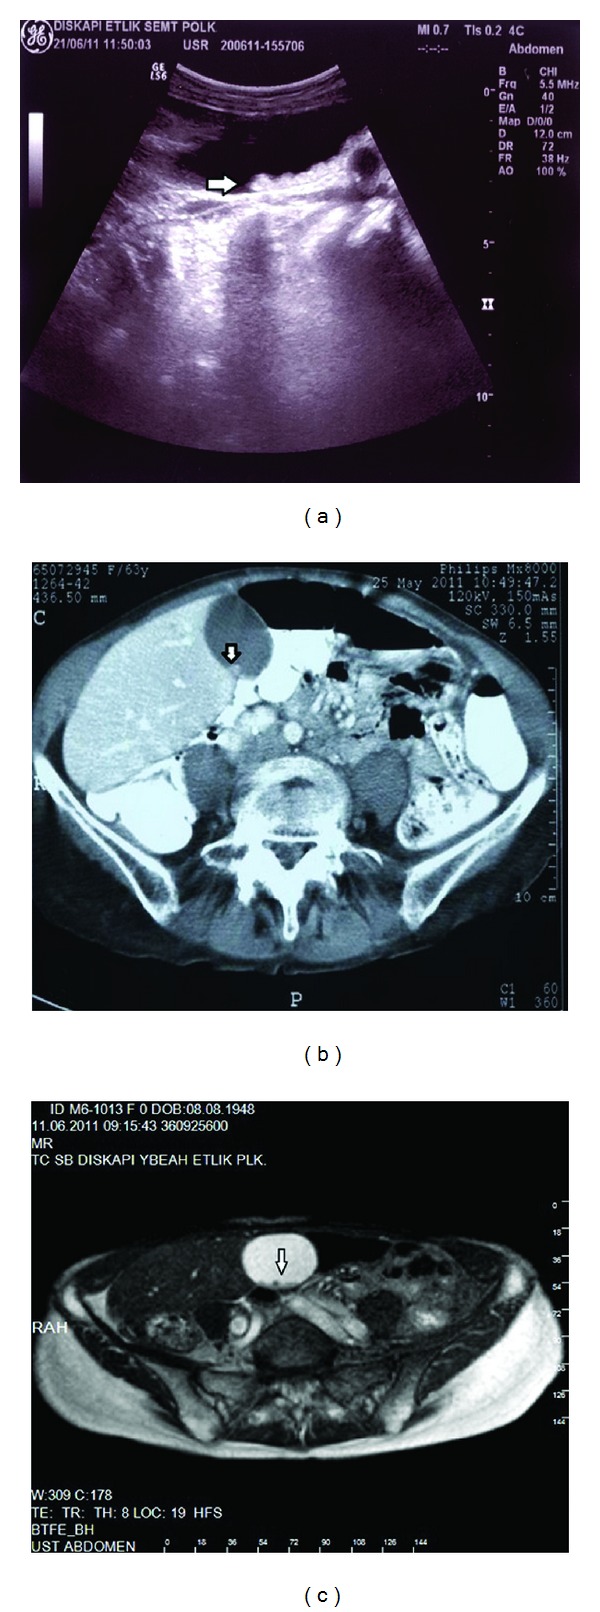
There was intraluminal sludge and gallstones of the GB (arrows). ((a) US, (b) CT, and (c) MRI findings).
